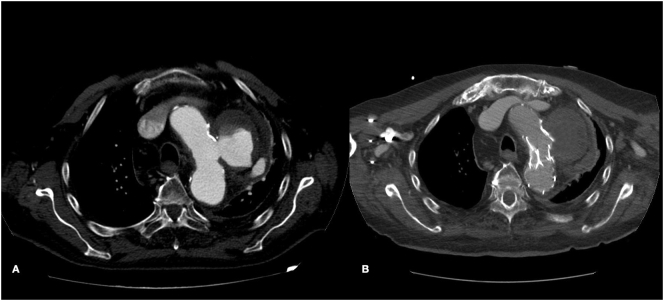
Preoperative CT Angiography (A) and postoperative CT Angiography (B).
